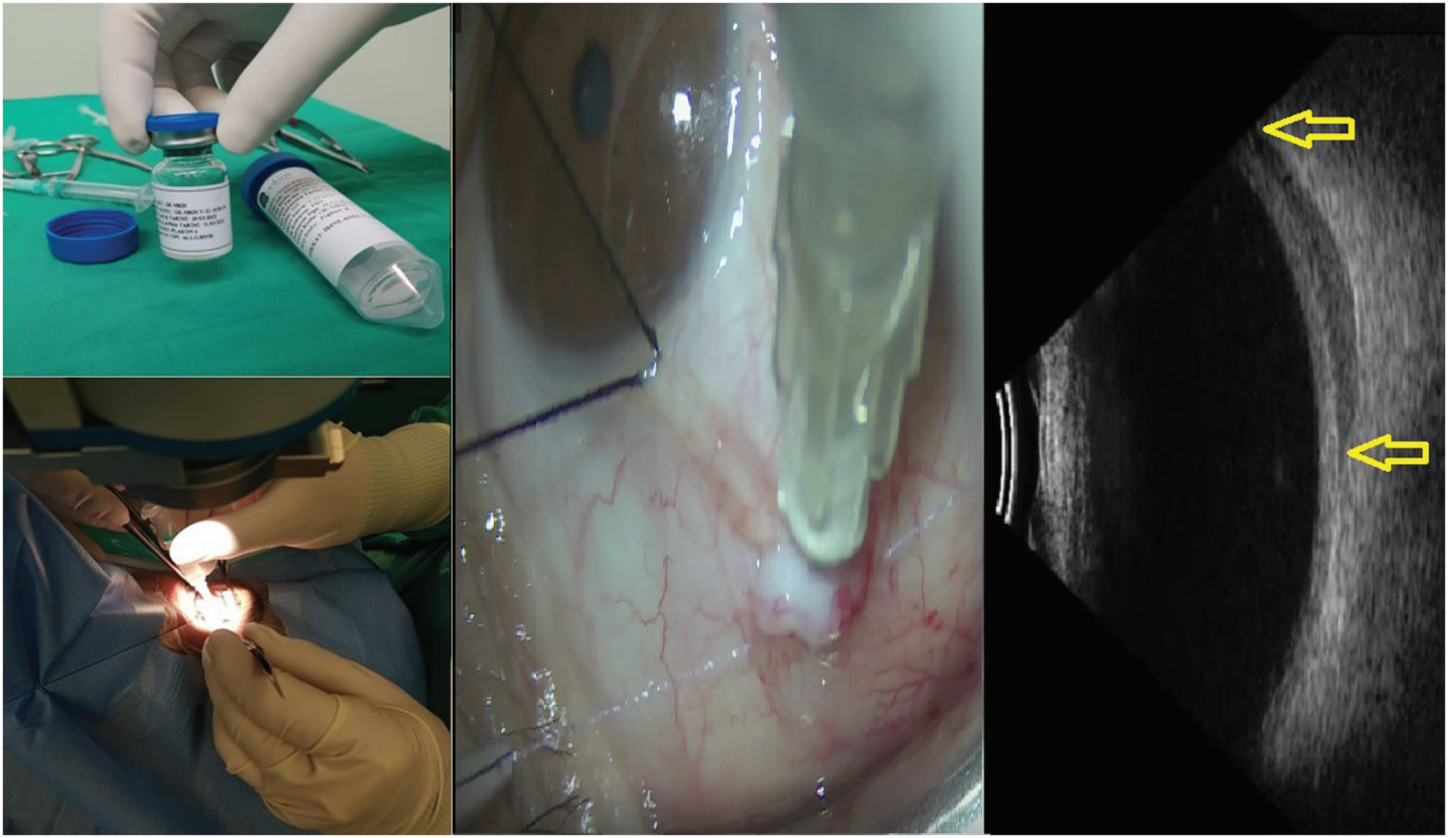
Deep subtenon injection of 5 000 000 WJ-MSC in 1.5 mL suspension and confirmation of inoculation site by orbital ultrasound.
